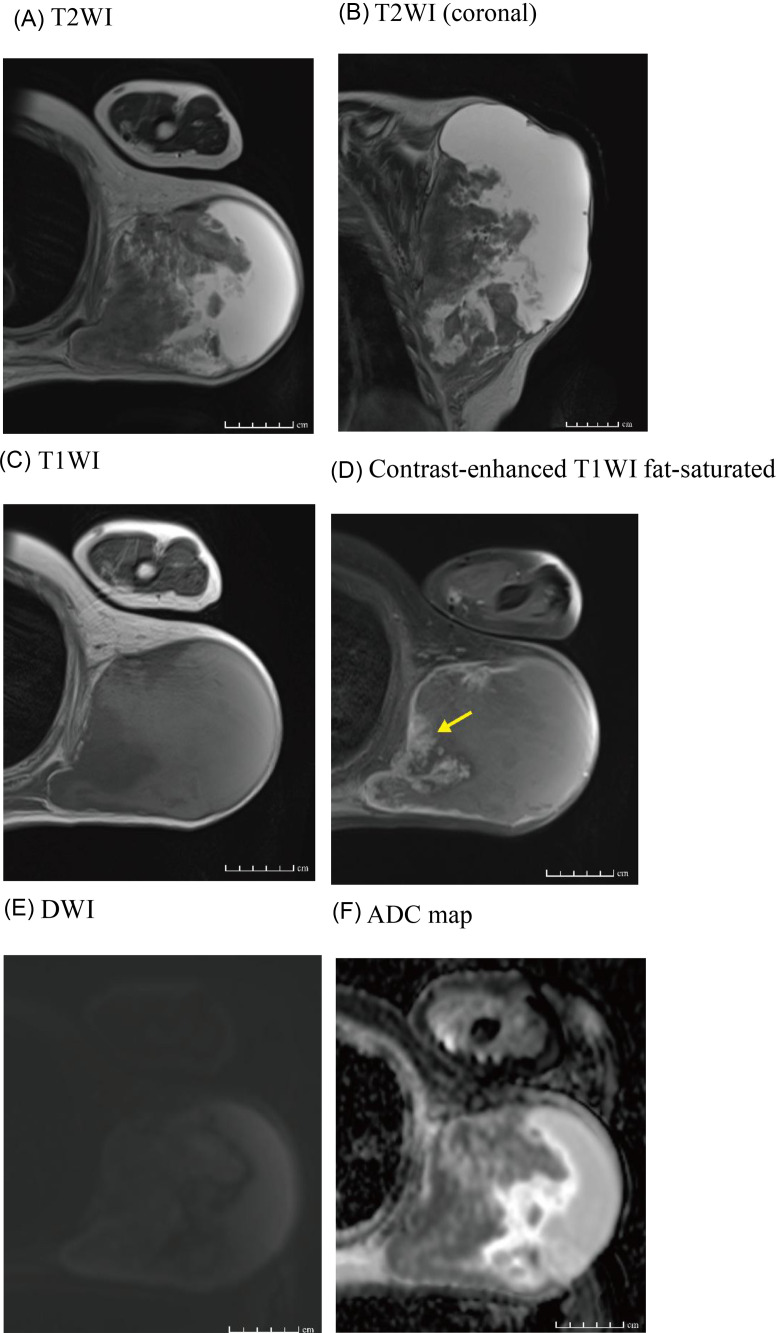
Case 4, a male in his 80s. Magnetic resonance imaging (MRI) is shown; (A) T2-weighted image (T2WI), (B) T2WI on coronal plane, (C) T1-weighted image (T1WI), (D) contrast-enhanced fat-saturated T1-weighted image (an enhancing mural component corresponding to the area of restricted diffusion is indicated by the yellow arrow), (E) diffusion-weighted image, and (F) apparent diffusion coefficient map.
